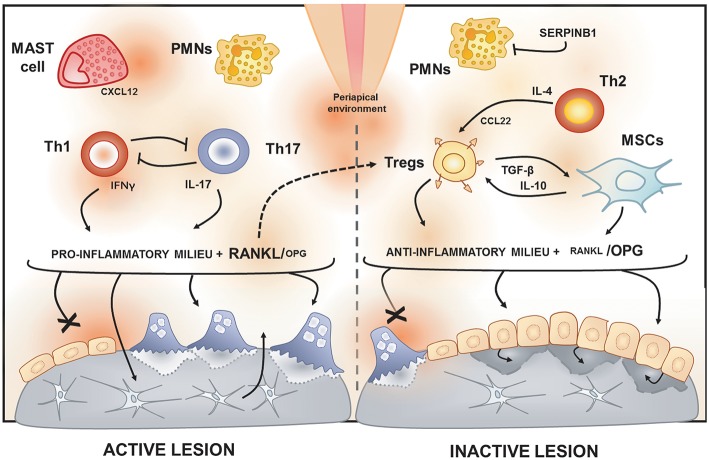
Immunological features of active and inactive periapical lesions. In the periapical environment, the presence of endodontic pathogens and its products (such as LPS) triggers the host inflammatory immune response. The analysis of gene expression signatures of periapical lesions allows for the discrimination of osteolytic activity status in “active” or “inactive” according to RANKL/OPG expression ratio. Active periapical lesions are characterized by high RANKL/OPG ratio is an association with a pro-inflammatory milieu, which includes a high level of IFN-g and IL-17. Distinct patterns of IFNg and IL-17 expression were described in periapical lesions, suggesting that Th1 and Th17 subsets, described to be mutually inhibitory, can drive apical periodontitis development independently. The presence of PMNs and mast cells also have been associated with lesion activity. Conversely, inactive lesions are characterized by a low RANKL/OPG ratio is an association with an anti-inflammatory milieu, which is supposed to involve a cooperative immunoregulatory network composed by Th2 and Tregs subsets, as well by MSCs. The prototypical Th2 cytokine IL-4 is described to induce the expression of CCL22, a main chemoattractant of Tregs. Noteworthy, Tregs hallmark products, such as IL-10 and TGF-β, are described to boost MSCs immunosuppressive properties. Interestingly, a recent study points to RANKL as an unexpected immunoregulatory feedback trigger via Tregs induction.
